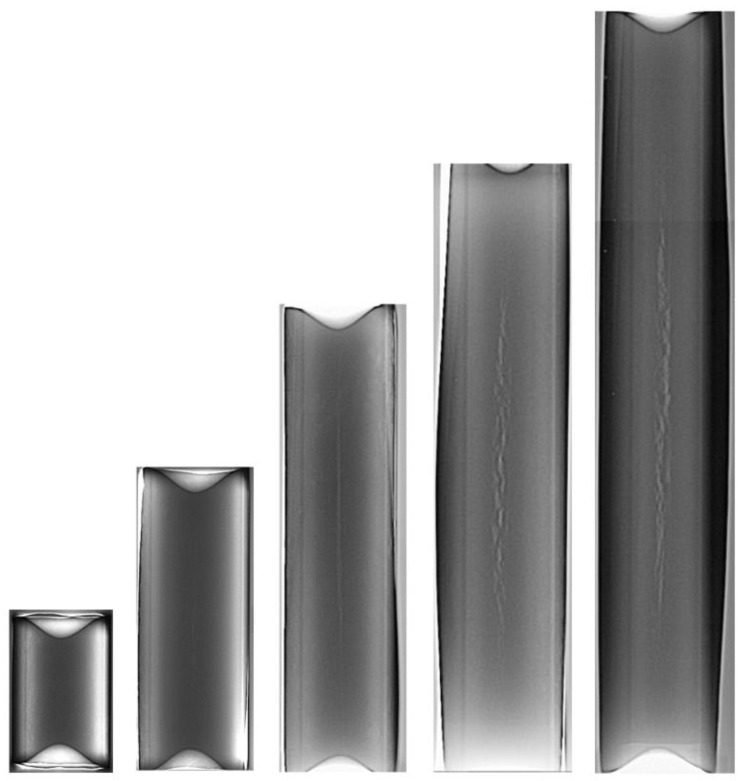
Exemplary roentgenograms of the rotationally compressed samples; dimensions of the samples—diameter 30 mm, initial length (from the left) 30, 60, 90, 120 and 150 mm.
